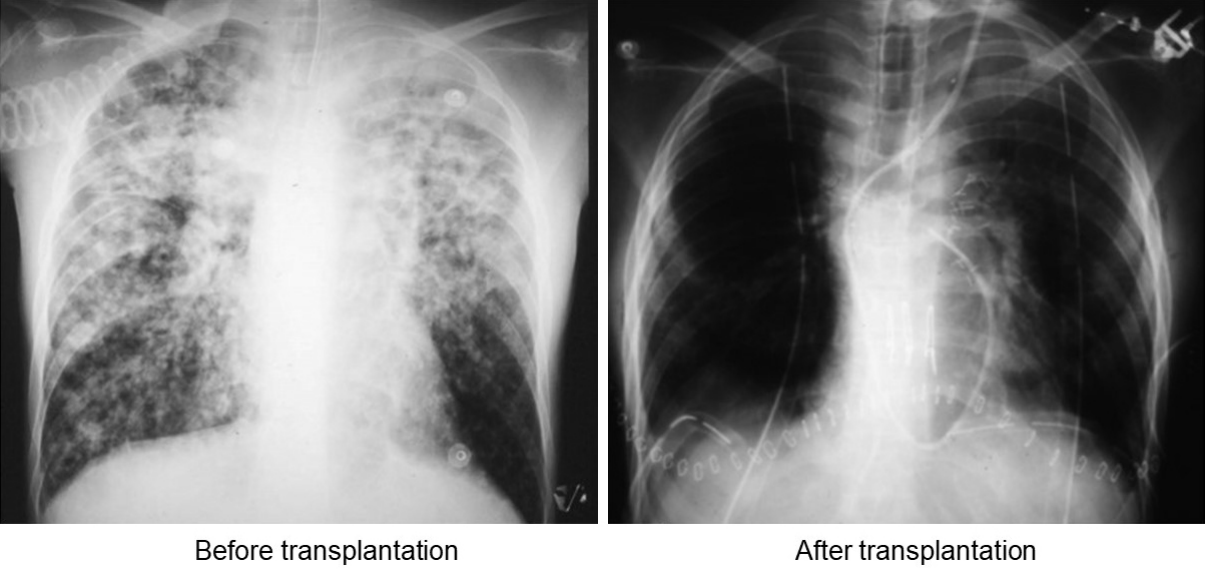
Chest X-ray before and after bilateral living-donor lobar lung transplantation Before transplantation, the patient required a ventilator due to Pseudomonas pneumonia. After transplantation, chest X-ray showed a significant improvement with clear translucency.
